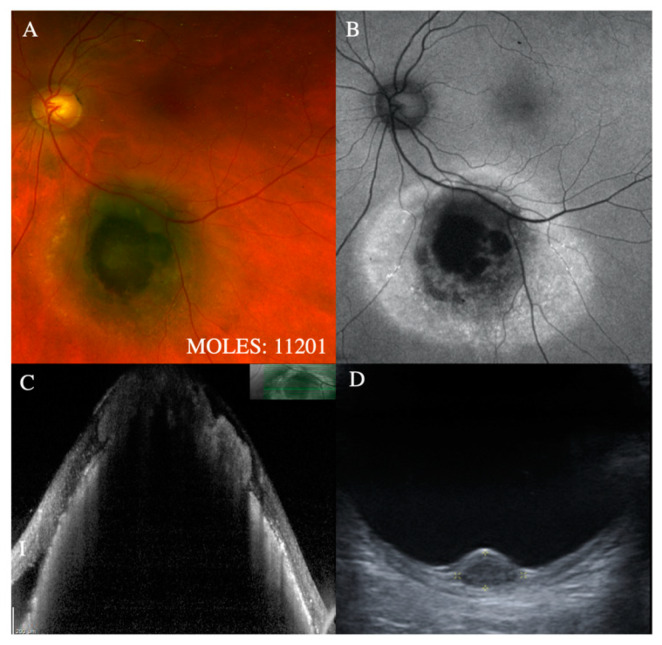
Representative cases demonstrating incipient mushroom shape (i.e., M = 1). (A) Fundus photographs showing focal atrophy of RPE, (B) highlighted as a well-defined region of hypo-autofluorescence (C) with corresponding area of RPE hyperplasia but (D) no evidence of a mushroom shape on B-scan ultrasonography (M = 1).
